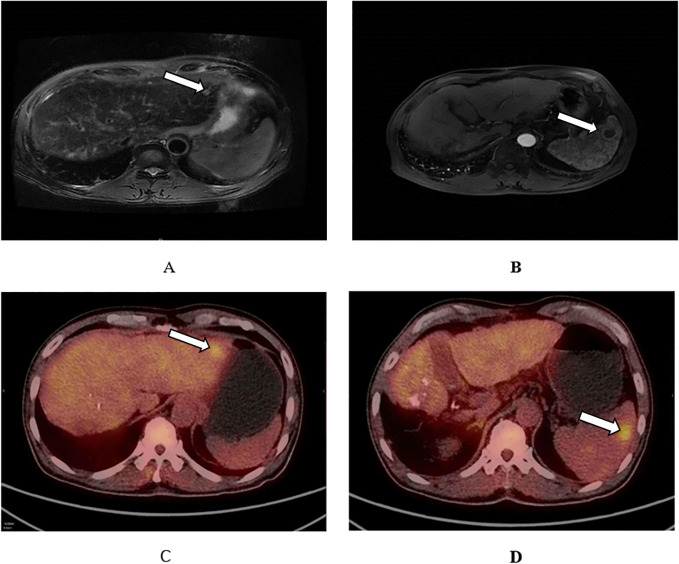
MRI and PET-CT images of metastatic tumors in the spleen. Panel (A) shows MRI. Plain T2-weighted images (T2WI). Panel (B) shows enhanced scan images. Panel (C) shows FDG hypermetabolic images of metastatic tumors in the left outer lobe of the liver. Panel (D) shows. The FDG hypermetabolic image of a splenic metastatic tumor. The location of the lesion has been marked with an arrow.
